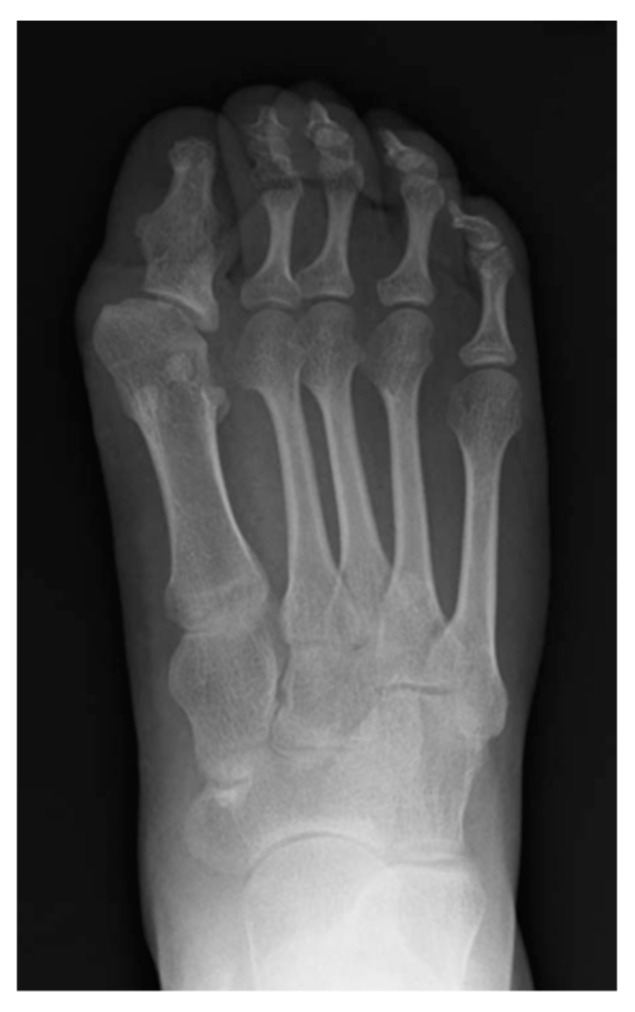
Anteroposterior radiograph of the right foot of 20-year-old female with FOP demonstrating medially deviated metatarsal bone and fused proximal and distal phalanges.
